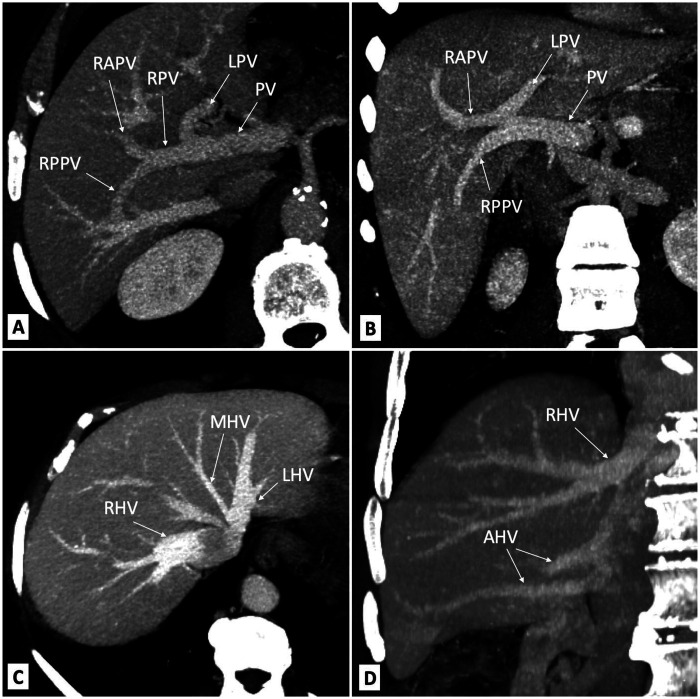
Portal vein trifurcation in liver computed tomography: (A) Nakamura's type A and Cheng's type I (bifurcation of the main PV into the LPV and RPV); (B) Nakamura's type B and Cheng's type II (trifurcation of the main PV into the LPV, RAPV, and RPPV; the common RPV is missing). Hepatic veins on computed tomography images: (C) main trunks of hepatic veins; (D) accessory hepatic veins. AHV, accessory hepatic vein; LHV, left hepatic vein; LPV, left portal vein; MHV, middle hepatic vein; PV, portal vein; RAPV, right anterior portal vein; RHV, right hepatic vein; RPPV, right posterior portal vein; RPV, right portal vein.
